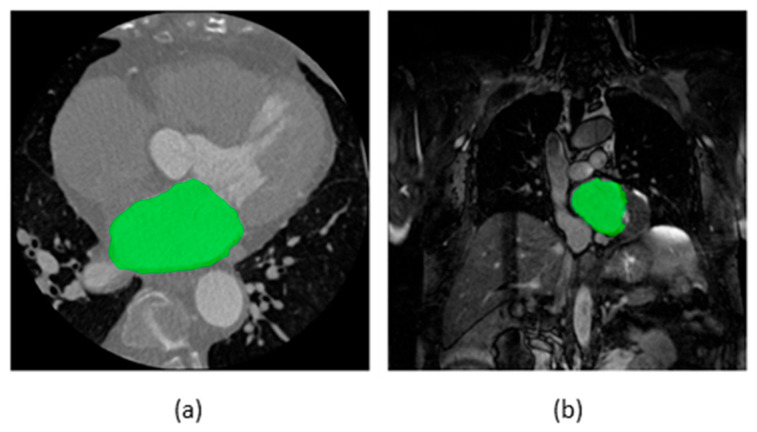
Segmentation maps of the left atrium superimposed on axial slices of (a) computed tomography and (b) magnetic resonance imaging scan. Source of scans: multi-modality whole-heart segmentation [21,22,23].
